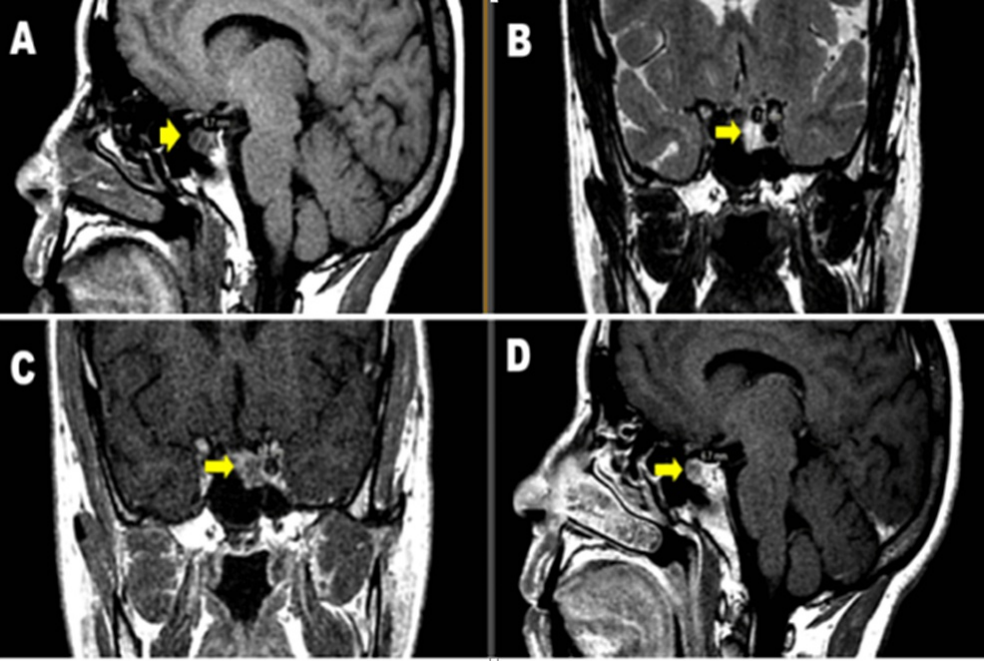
MRI images: sagittal T1 (A), coronal T2 (B), coronal T1 C + (C), and sagittal T1 C + (D) showing a 7-mm anteropituitary microadenoma in hyposignal T1, hypersignal T2 (Department of Radiology CHU Hassan II Fez).
